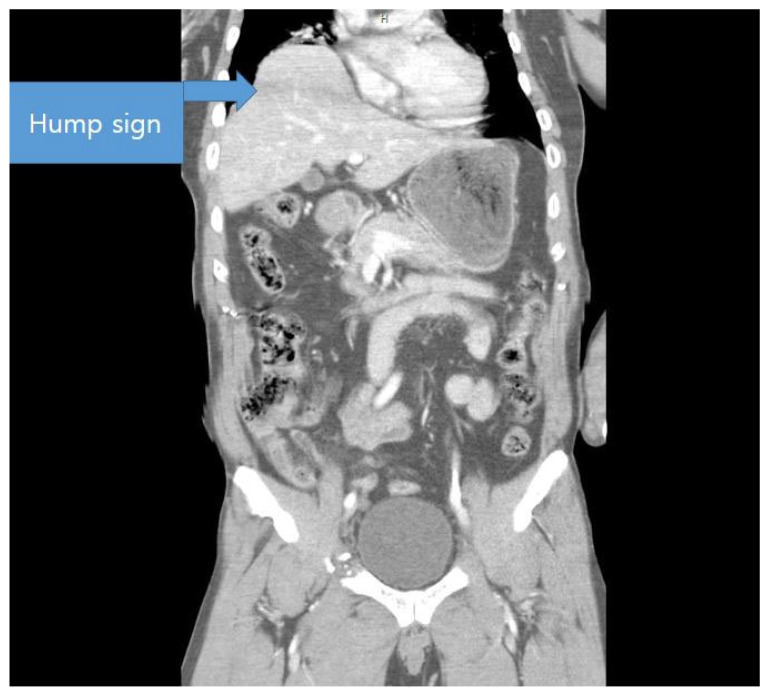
Hump sign. Computed tomography image showing partial herniation of the liver into the thoracic cavity, indicating right-sided diaphragmatic injury.
